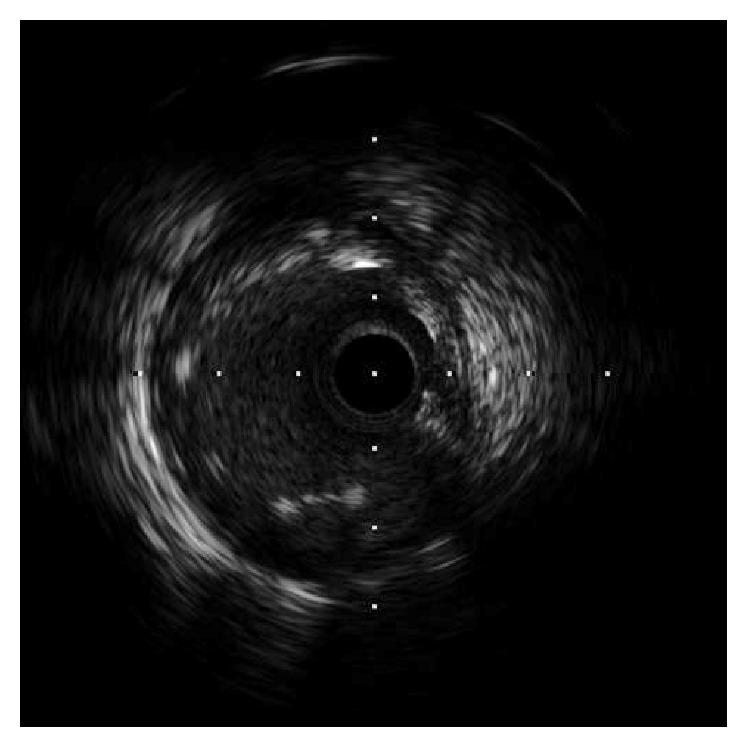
Intravascular ultrasound imaging revealed incomplete apposition of the stent struts in the proximal edge.
